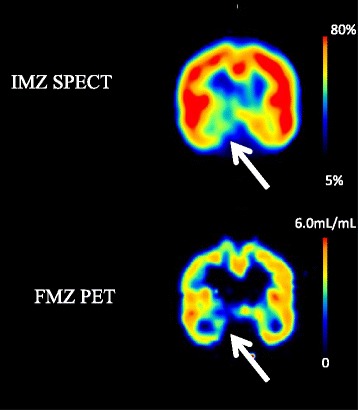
Case 1 (Patient No. 1): The patient was a 28-year-old male who was injured in a car accident 1 year previously. No abnormalities were found at the time of injury but he complained of irritability and poor concentration after the injury. Microbleeds in the right parietal lobe and left temporal lobe were observed on MRI images in the chronic phase. FMZ BP was low inside the right temporal lobe. The low uptake level inside the right temporal lobe was also observed by IMZ SPECT
